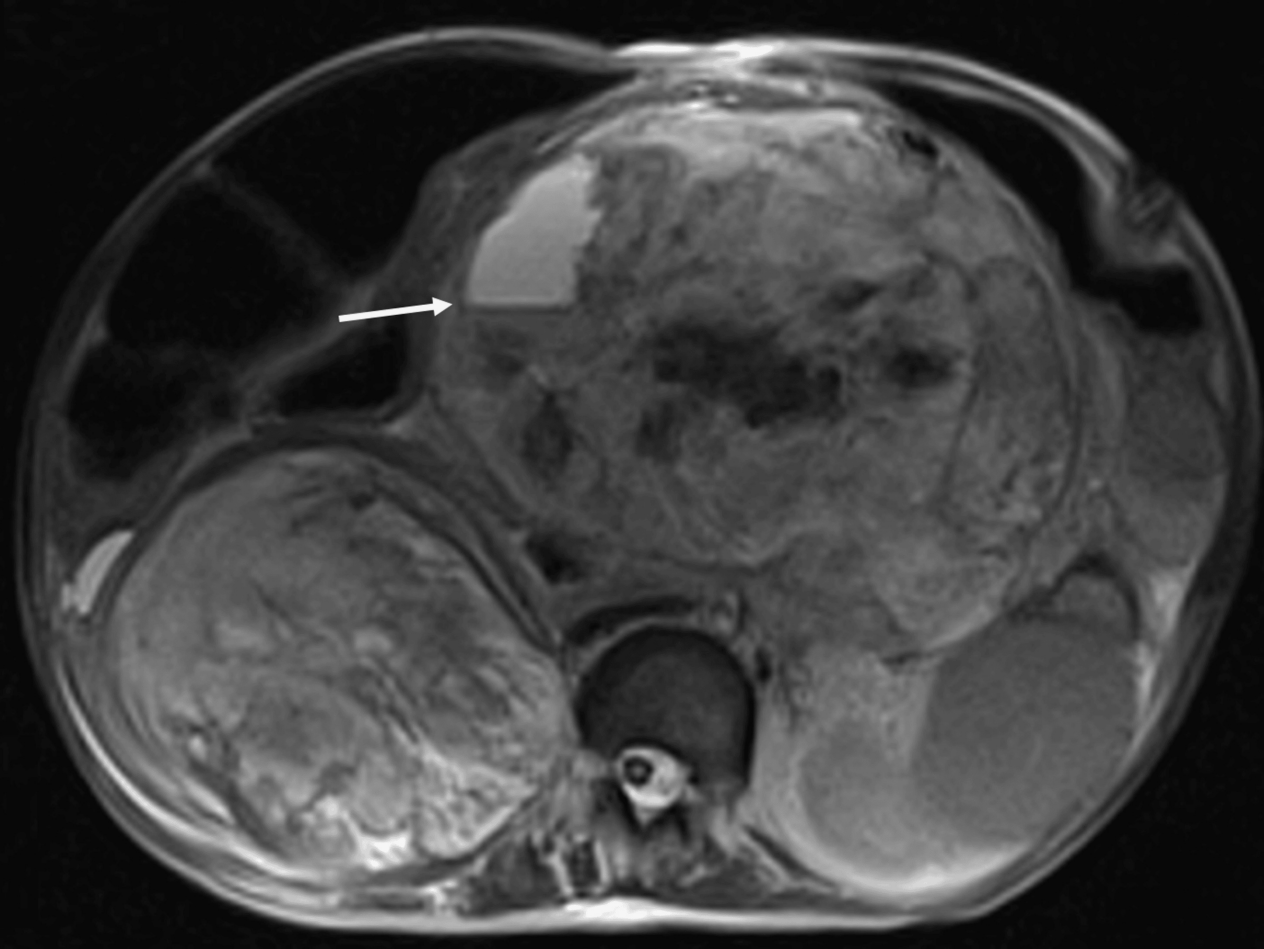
T2-weighted fat-saturated axial image of the abdomen demonstrating fluid levels within the mesenteric mass.
